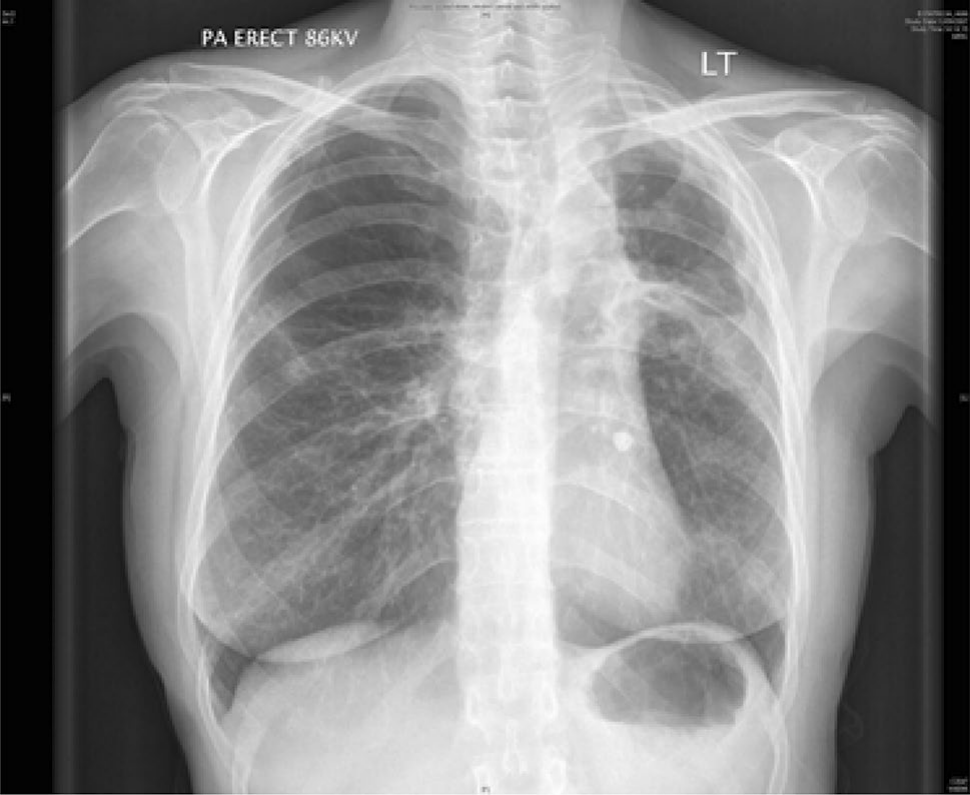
Chest X-ray of an ex-smoker (30 pack-years) patient with COPD and emphysema who developed increasing frequency of respiratory infections. Imaging shows upper lobe fibrosis with traction bronchiectasis and a positive *Aspergillus* IgG antibody test. A large cavity is visible occupying the whole left upper lobe
